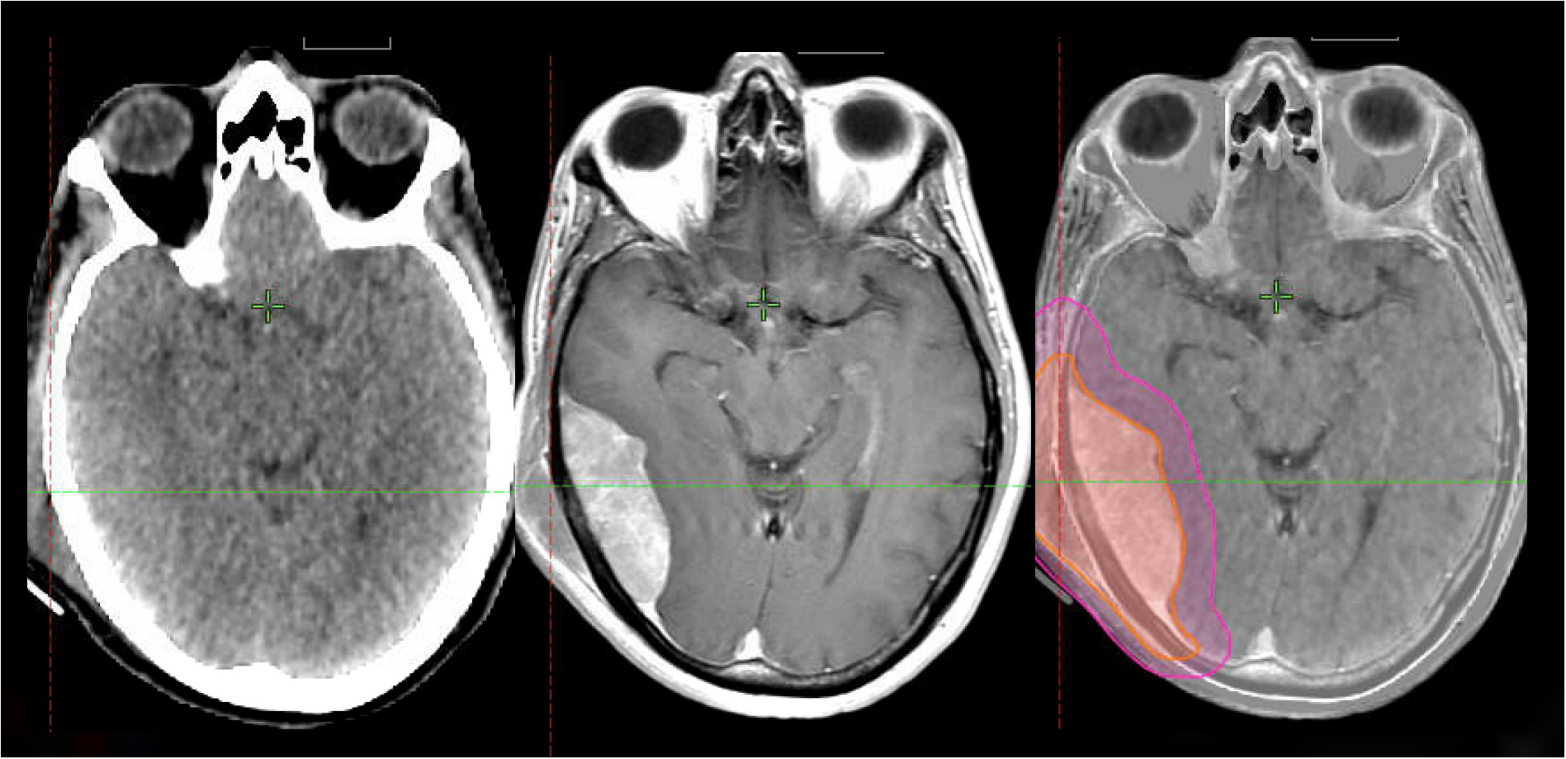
50-year-old man with R-ISS 1 multiple myeloma with a right scalp myelomatous lesion status-post-transplant while on maintenance therapy. MRI showed osseous and dural involvement. He received 24 Gy in 12 fractions with VMAT and achieved a complete response.
